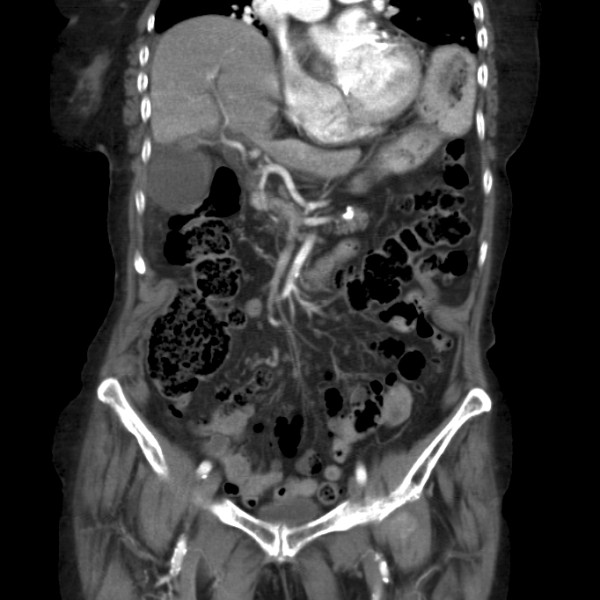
CT scan of the abdomen. Coronal view of the abdomen, acquired using a computed tomography scan with intravenous contrast, at presentation of the patient in the emergency department. The gallbladder is hydropic, with a thickened wall and positioned adjacent to the lateral abdominal wall. No gallstones are visible. Some isolated free fluid is seen between the right liver lobe and the diaphragm, as well as some fluid and fatty infiltration around the gallbladder. Radiological findings are suggestive of an acalculous cholecystitis.
